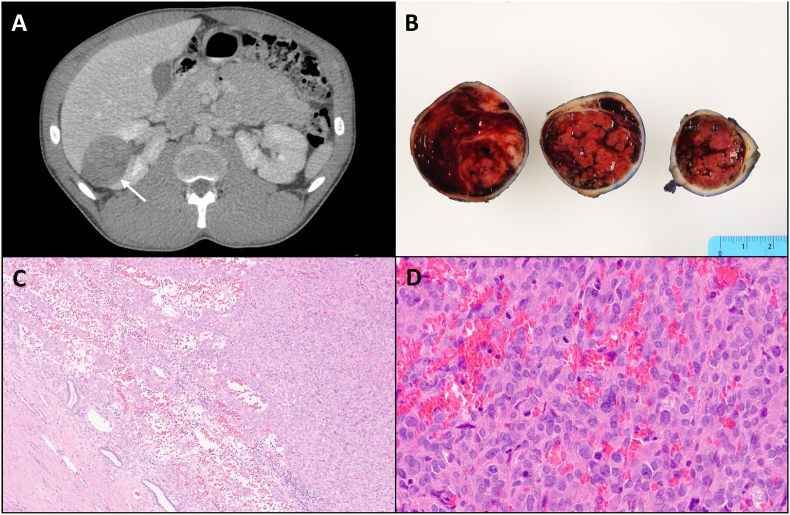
An axial computed tomography image with intravenous contrast demonstrating a solid mass (white arrow) in the lateral mid pole of the right kidney (A). Gross image of the tumor showing well-defined borders with a fleshy, hemorrhagic cut surface (B). Tumor borders are relatively sharp with occasional entrapped renal tubules at the periphery (C). The tumor is composed of ovoid to spindle cells with moderate nuclear pleomorphism, predominantly eosinophilic cytoplasm, and intimately associated with surrounding delicate vasculature. Mitotic figures are readily apparent (D).
